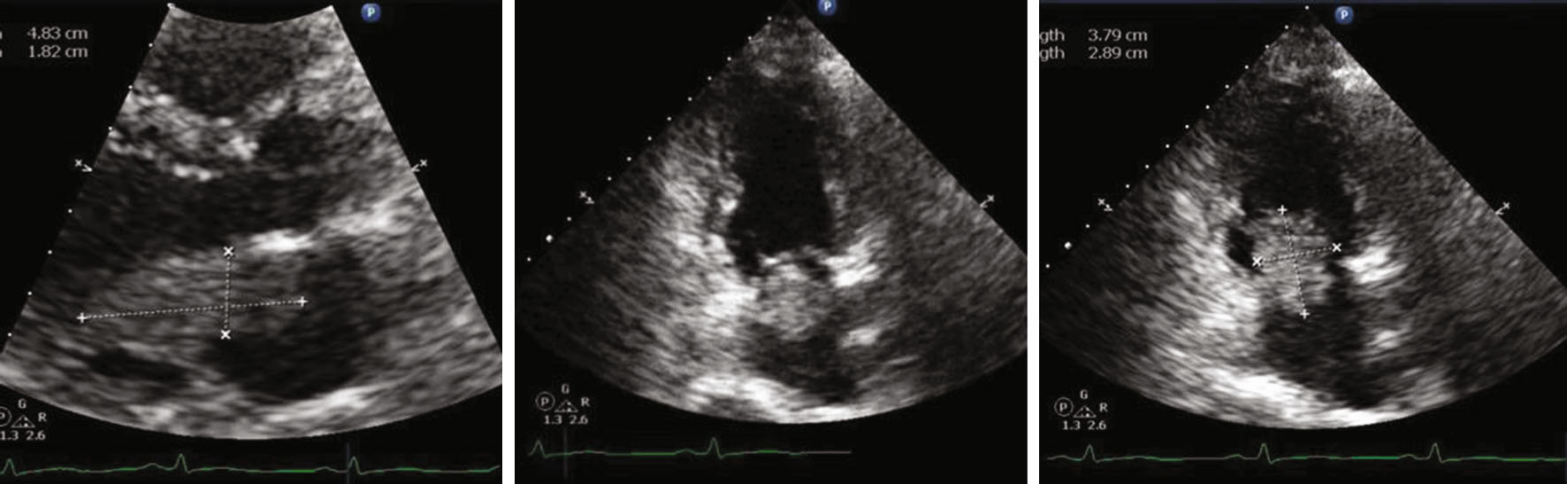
Transthoracic echocardiogram. There is a large, 5.7 *cm* (*L*) × 1.9 *cm* (*W*), mobile mass on the left side of the interatrial septum; the appearance is consistent with myxoma (echocardiographic contrast reveals vascularity of the mass). The mass travels along with the anterior mitral leaflet and plops in and out of the left ventricle. Transvalvular velocity of the mitral valve is within the normal range, and there is no evidence of stenosis. Left ventricular ejection fraction was in the range of 55% to 60%.
